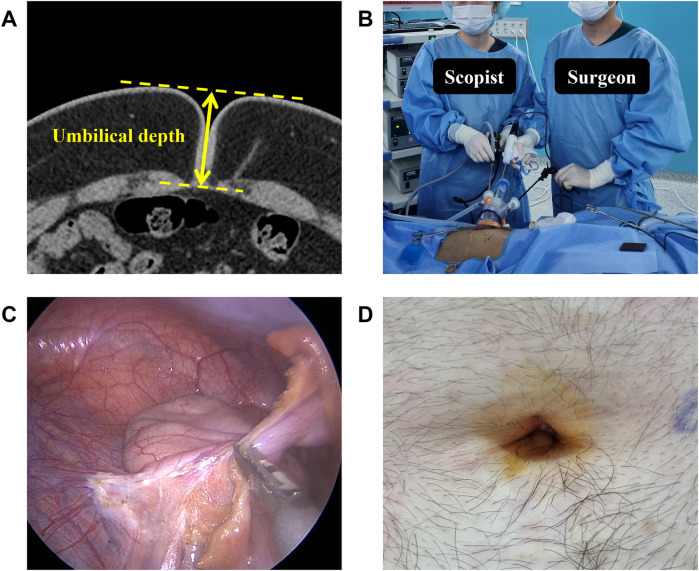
Single-port laparoscopic appendectomy. (A) Umbilical depth was measured in preoperative CT. (B) Operator stood to the left of the scopist and used only straight, rigid laparoscopic instruments. (C) Ultrasonic surgical devices were used to resect the mesoappendix and ligate the appendiceal artery. (D) Umbilical wound on postoperative day 3.
